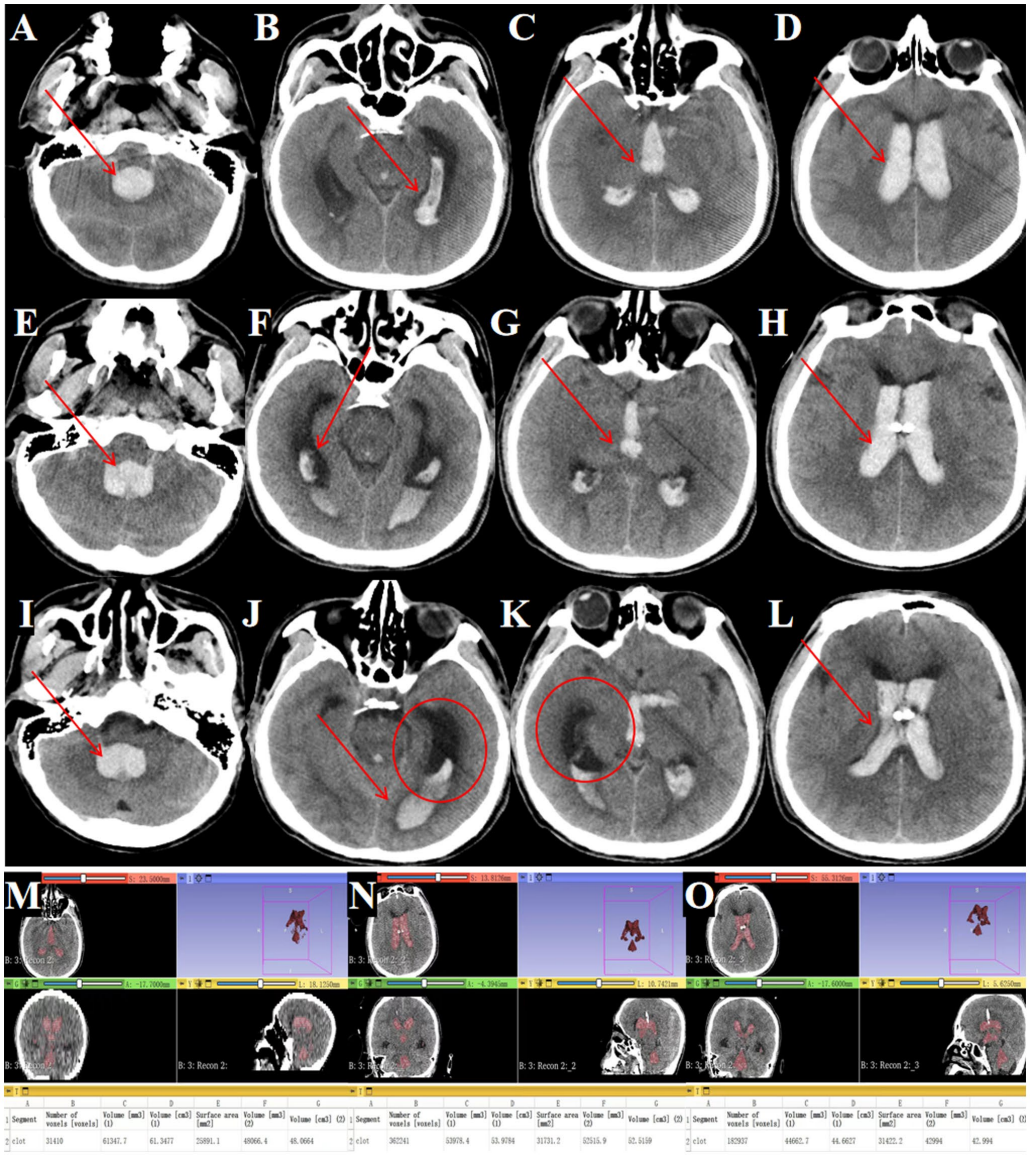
Outcomes of traditional EVD in complete IVH casting. (A–D) Admission head CT showing complete IVH casting (→ arrows mark hemorrhage in the fourth, third and lateral ventricles). (E–H) Postoperative CT after bilateral EVD (→ arrows highlight residual hematoma in all ventricles). (I–L) Day 3 post-EVD CT demonstrating ongoing IVH casting (→ arrows denote unresolved hemorrhage; ◯ circles confirm worsening ventricular dilation). (M) Preoperative hematoma volume: 61.3 mL. (N) Postoperative hematoma volume reduced to 53.9 mL. (O) Day 3 post-surgery hematoma volume: 44.6 mL.
